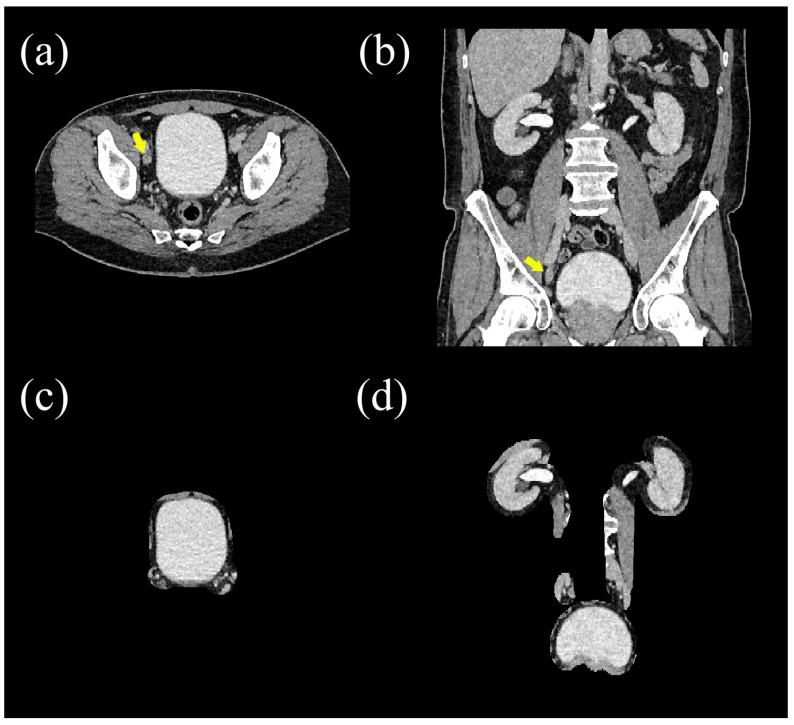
Example of an incidental finding (enlarged right para-iliac lymph node, indicated with an arrow on unmodified axial CT (a) and coronal CT (b)) which was successfully concealed on focused view CTU (c,d), allowing for prospective follow-up in a trial investigating the relevance of incidentalomas.
